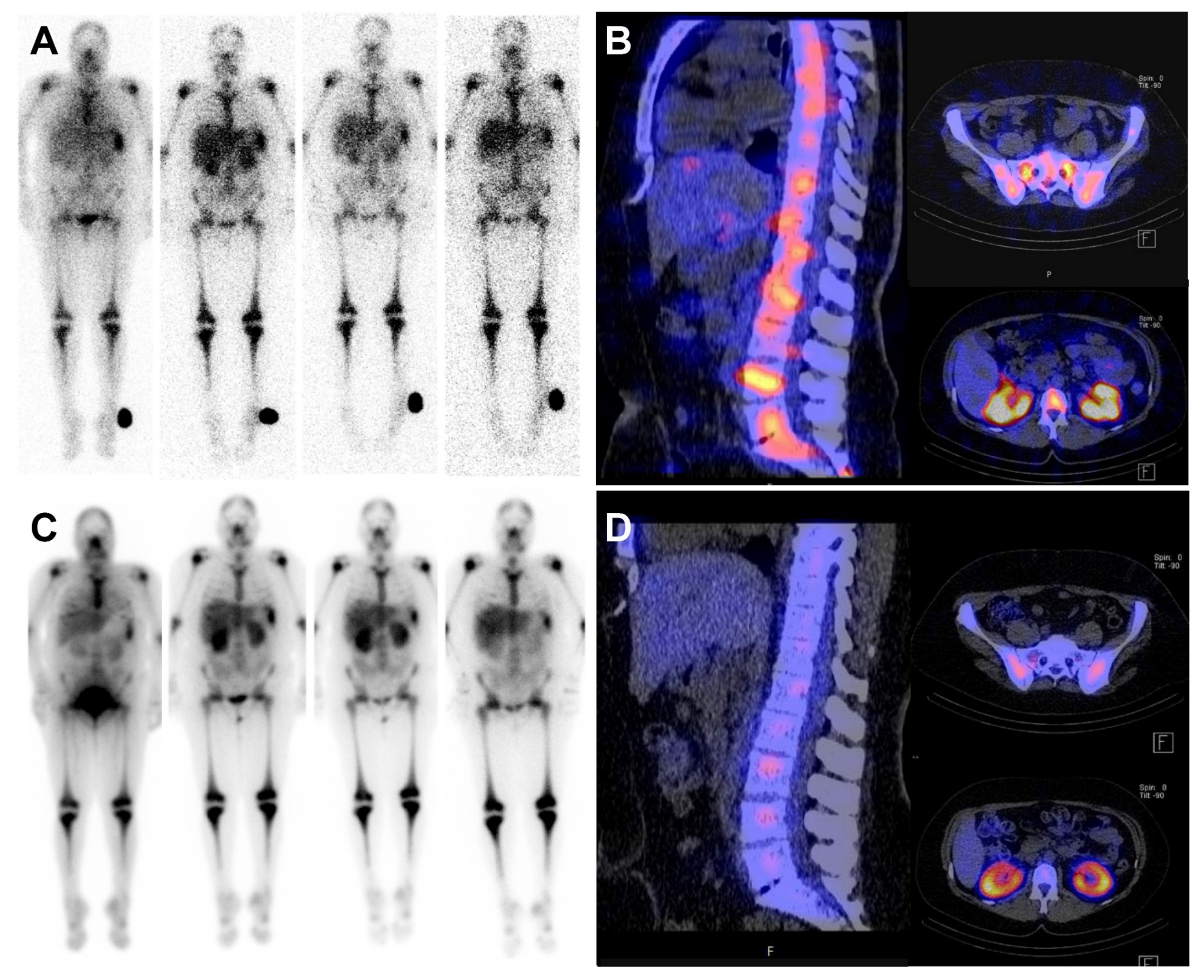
Pre- and post-therapeutic dosimetry. Pre- and post-therapeutic scintigraphy and SPECT/CT imaging of a 47 year old patient with refractory AML. Pre-therapeutic whole-body scintigraphy at 1 hour post injection (p.i.); 22h p.i.; 48h p.i. and 6 days p.i. (A, from left to right) for dosimetry including fused SPECT/CT (B). Post-therapeutic whole-body scintigraphy at 2 h, 24 h, 48 h and 6 d after administration of 14.4 Gbq [^177^Lu]Pentixather (C) and fused SPECT/CT (D).
